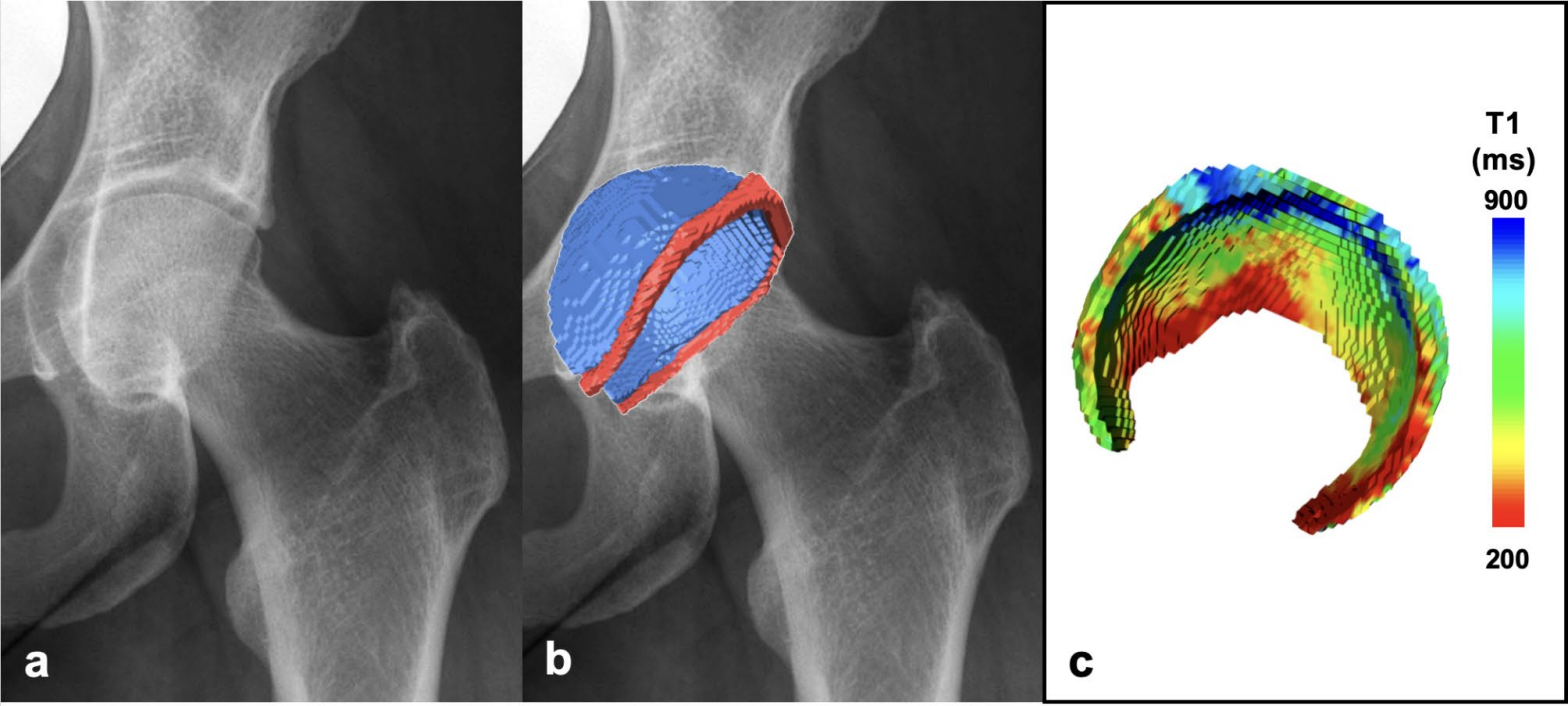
Patient example. (a) Antero-posterior radiograph of a 31 year old patient with pincer morphology. (b) 3D morphologic model of hip cartilage and labrum demonstrate a small labrum with a volume of 1043 mm^3^, (cartilage volume = 5438 mm^3^, relative labrum volume = 19%), indicating labrum hypotrophy as a result of global acetabular overcoverage. (c) 3D cartilage model with color coded T1 mapping demonstrates the typical pincer wear pattern of central joint degeneration. 3D models were visualized using a custom-made software application for automatic segmentation and visualization^8^. damage.
